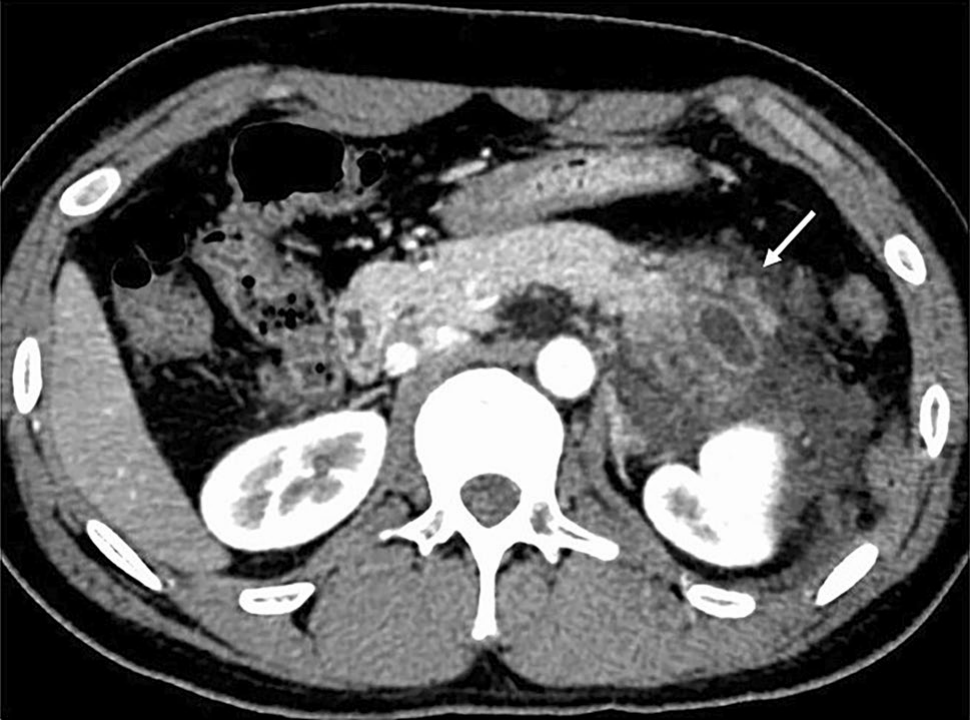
Computed tomography at the time of transfer to our hospital. Focal enlargement of the pancreatic tail, increased fat tissue density, and fluid accumulation in the surrounding area are observed (arrow). An oval cystic lesion covered with a thick capsule is observed within the swollen pancreatic tail
